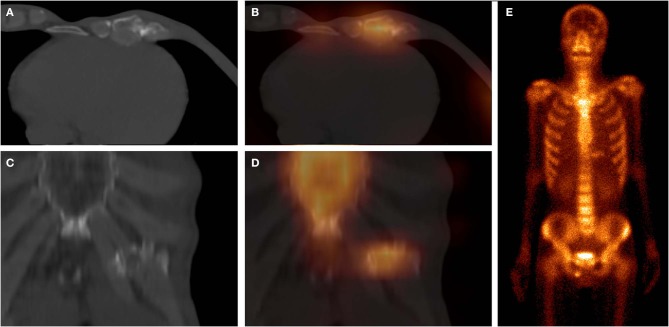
These images were obtained from a 64-year-old patient of CG, who underwent chemotherapy and surgery for esophageal cancer 6 months ago. ^99m^Tc-MDP SPECT/CT axial images (A, CT; B, fusion), coronal images (C, CT; D, fusion), and WBS image (E, anterior) demonstrated moderate-uptake tracer activity in the sixth and seventh costal cartilages, with irregular calcification and local deformation. The pathological findings confirmed the diagnosis of costal chondritis.
